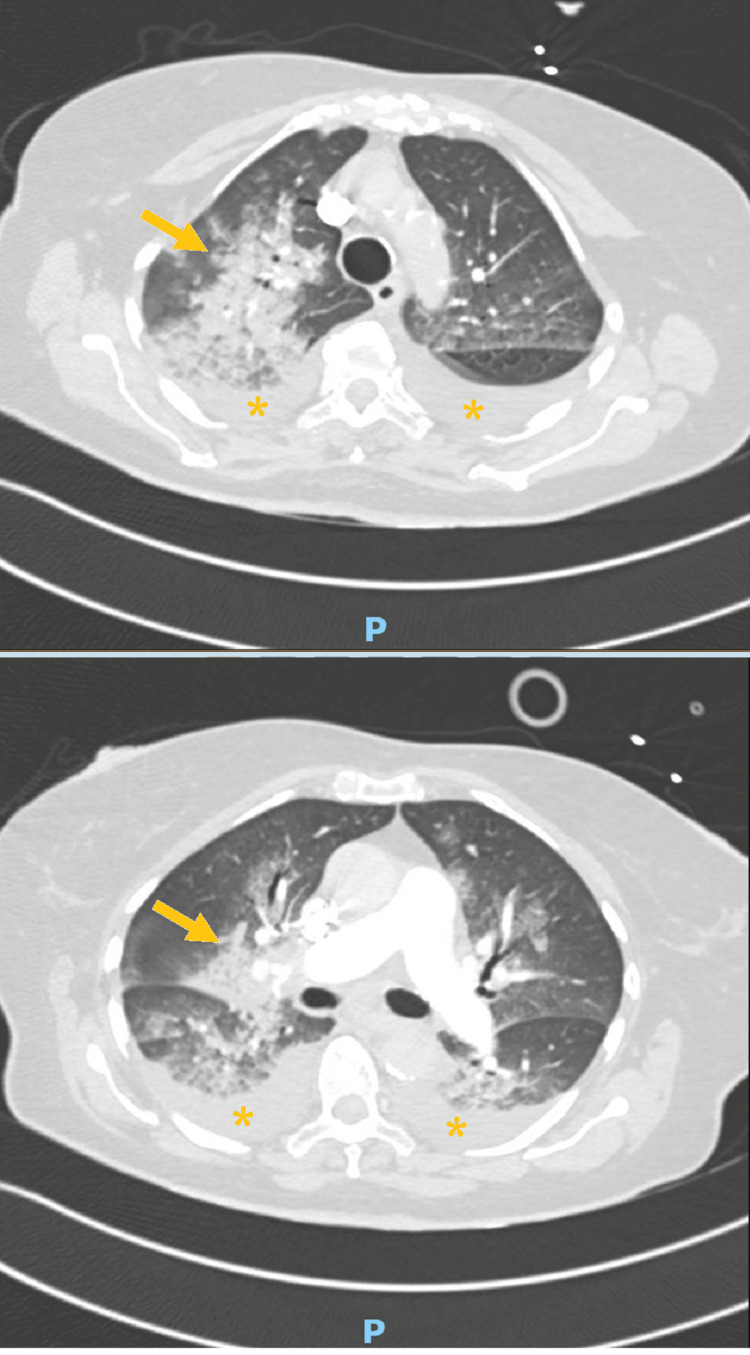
Chest computed tomography angiography Arrows: consolidation in the apical segment of the right upper lobe. Asterisks: minor bilateral pleural effusion.
